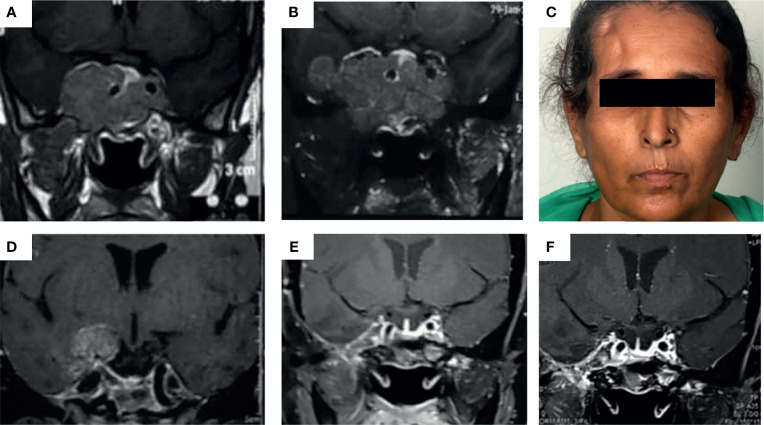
(A–E) Images depicting the baseline MRI of a patient presenting with headache and secondary amenorrhea due to an invasive macroprolactinoma (A) which was dopamine agonist resistant. She underwent transsphenoidal surgery elsewhere with significant tumor residue (B), necessitating redo surgery by the transfrontal route at our institute (right pterional craniotomy) (C). Due to persistent right temporal lobe residual mass (D), the patient was managed with intensity-modulated radiotherapy (50 Gy over 28#) along with initiation of temozolomide therapy at 150 mg/m^2^ dose for the first month followed by 200 mg/m^2^ dose in 5/28 day cycles, for a total of 9 cycles. This led to almost complete resolution of the tumor in the patient as well as normalization of serum prolactin (E) which was persistent even after 2 years of follow-up (F).
